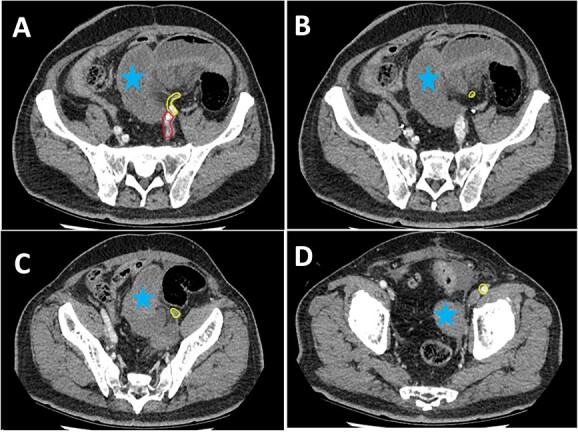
Series of axial images of initial CT progressing from most superior (image A) to inferior (image D). The EIA has been highlighted in yellow from immediately inferior to the bifurcation of the common iliac artery. The internal iliac artery can be seen in red in image A. Images B and C demonstrate a collapsed EIA between dilated loops of small bowel suggesting involvement in the internal hernia. Image D demonstrates opacification of the EIA indicating flow distal to the internal hernia. Dilated loops of bowel have been marked with a blue star.
